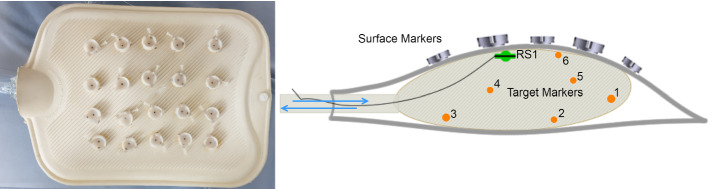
Left: Respiratory model with the fixed SHs. Right: Inside of the model. To place the markers in the balloon, the balloon is turned inside–out and 6 X-Spot CT skin markers ($${\oslash }$$ 1.5 mm, lead-free metallic pellet), 1 titanium Rhinospider ball ($${\oslash }$$ 4 mm) isocentrically carrying a cylindrical 5D magnetic sensor ($${\oslash }$$ 0.5 mm, L 8 mm), are glued on the inner wall of the balloon (maximum inflation $${\oslash }$$ 120 cm). Thereafter the balloon is turned outside–in. For inflating with a water blaster ($$82\times 5\times 15$$ cm), a flexible silicone tube ($${\oslash }$$ 20 mm, L 200 cm) is connected to the balloon
